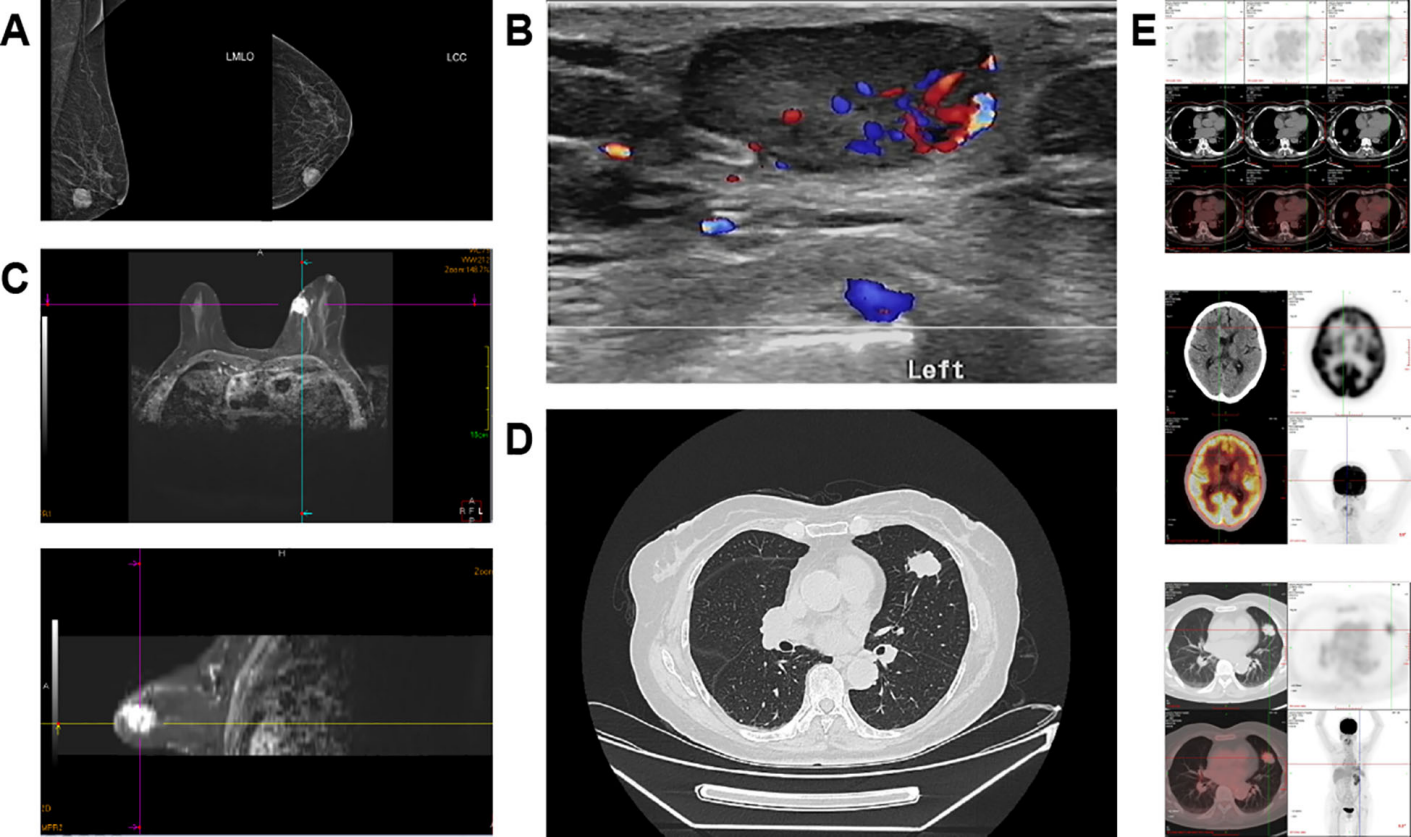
The imaging examination results of the patient. (A) Mammography of the breast. (B) Breast magnetic resonance imaging (MRI). (C) Color Doppler ultrasound of the breast. (D) Thoracic-abdominal computed tomography (CT) at the level of the lungs. (E) Positron emission tomography/computed tomography (PET/CT).
